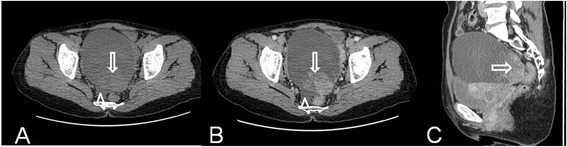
Ovarian clear cell carcinoma in a 49-year-old woman. Axial precontrast MSCT image (A) shows a mostly cystic, unilocular, and oval-shaped mass with smooth margin and irregular solid protrusion (arrow). Axial (B) and sagittal (C) contrast-enhanced CT images show obviously heterogeneous enhancement of the solid component in the tumor (arrow). Note the minimal fluids (arrowhead).
